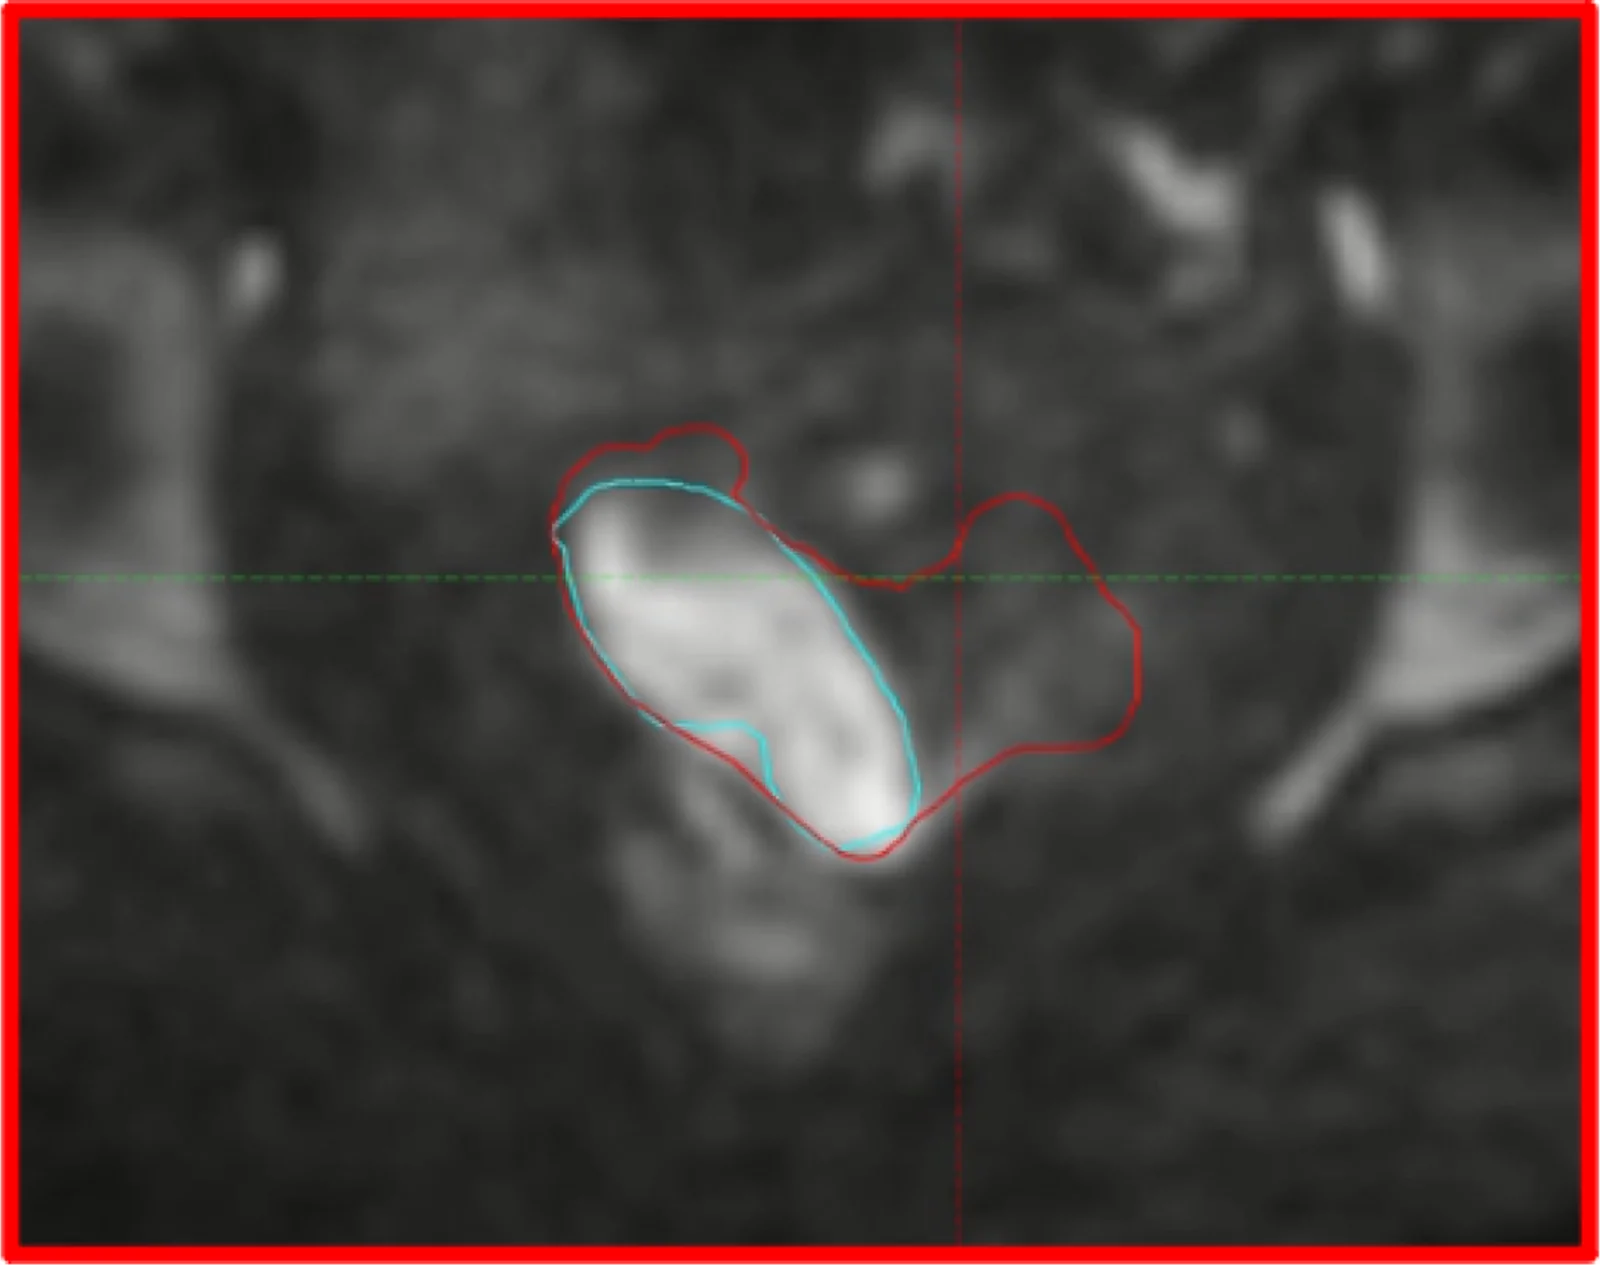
a. Figure showing T2 weighted MRI with GTV_T2 in red outline, depicting bulky tumor at the posterior lip of cervix. (For interpretation of the references to colour in this figure legend, the reader is referred to the web version of this article.) b. Figure showing the GTV at pre_EBRT; superimposed GTV_T2 in red outline and GTV_T2/DWI in cyan outline that depicts no diffusion restriction in the left half. (For interpretation of the references to colour in this figure legend, the reader is referred to the web version of this article.)
